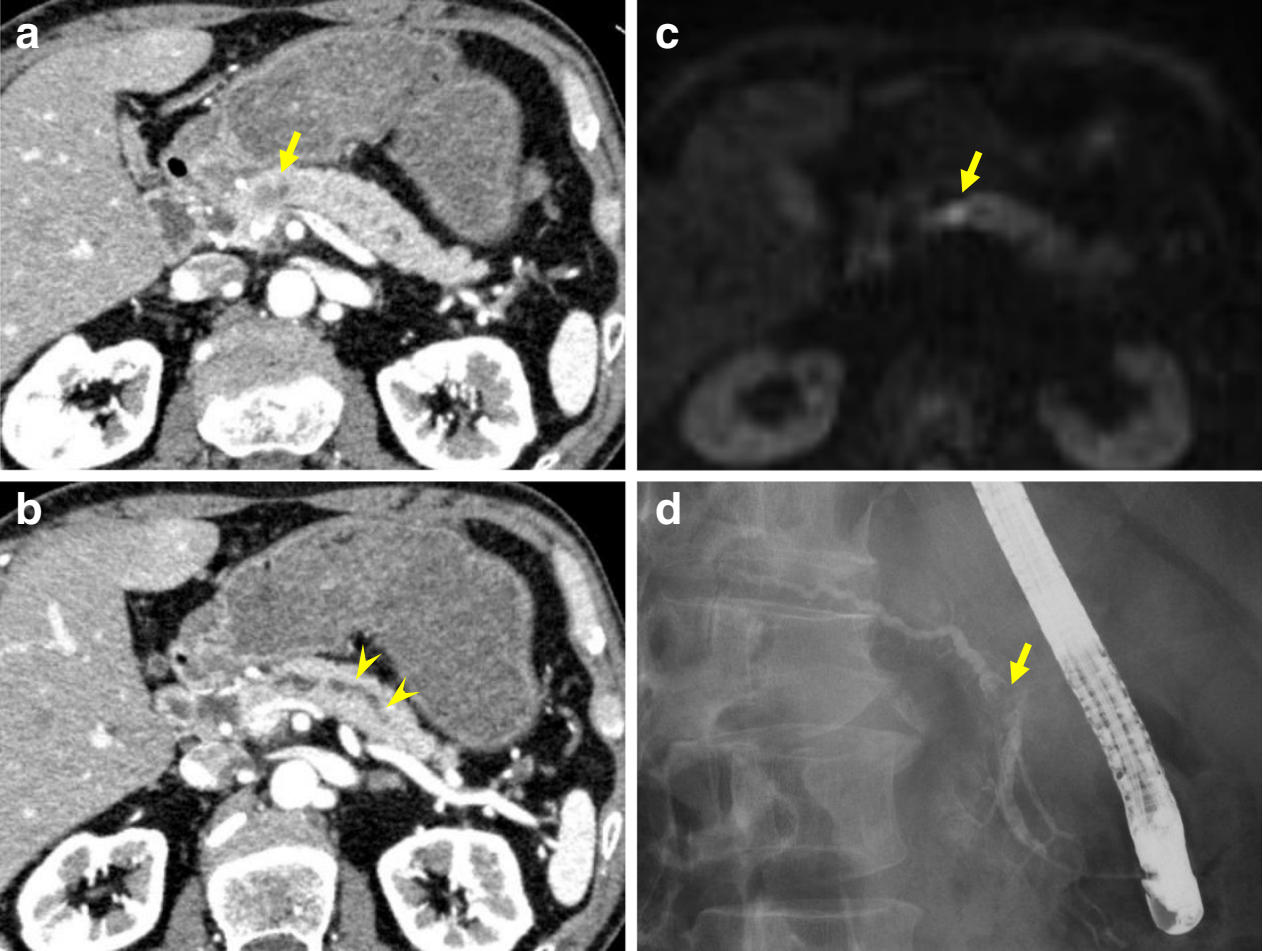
Preoperative image findings in the first surgery. a, b CT showed a low-contrast tumor approximately 1 cm (arrow) in size and dilatation of the upstream main pancreatic duct (arrowhead). c MRI revealed a tumor showing a high signal by DWI at the stenosis of the pancreatic duct. d ERCP showed an irregular defect in the main pancreatic duct
